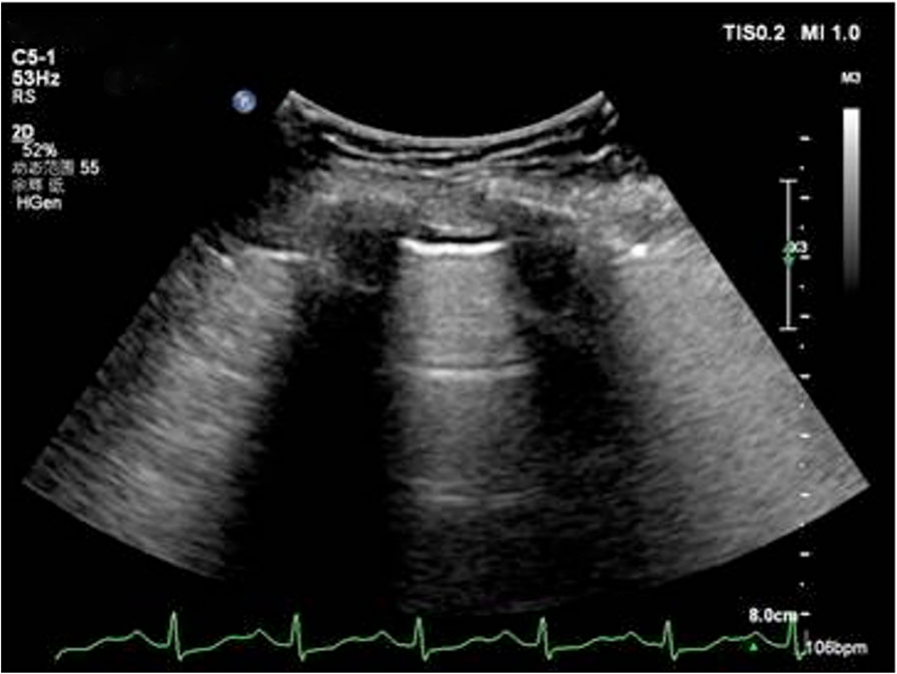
Sonographic signs of lung Normal pattern: sliding sign plus A-lines or less than three B-lines in a longitudinal plane between two ribs
